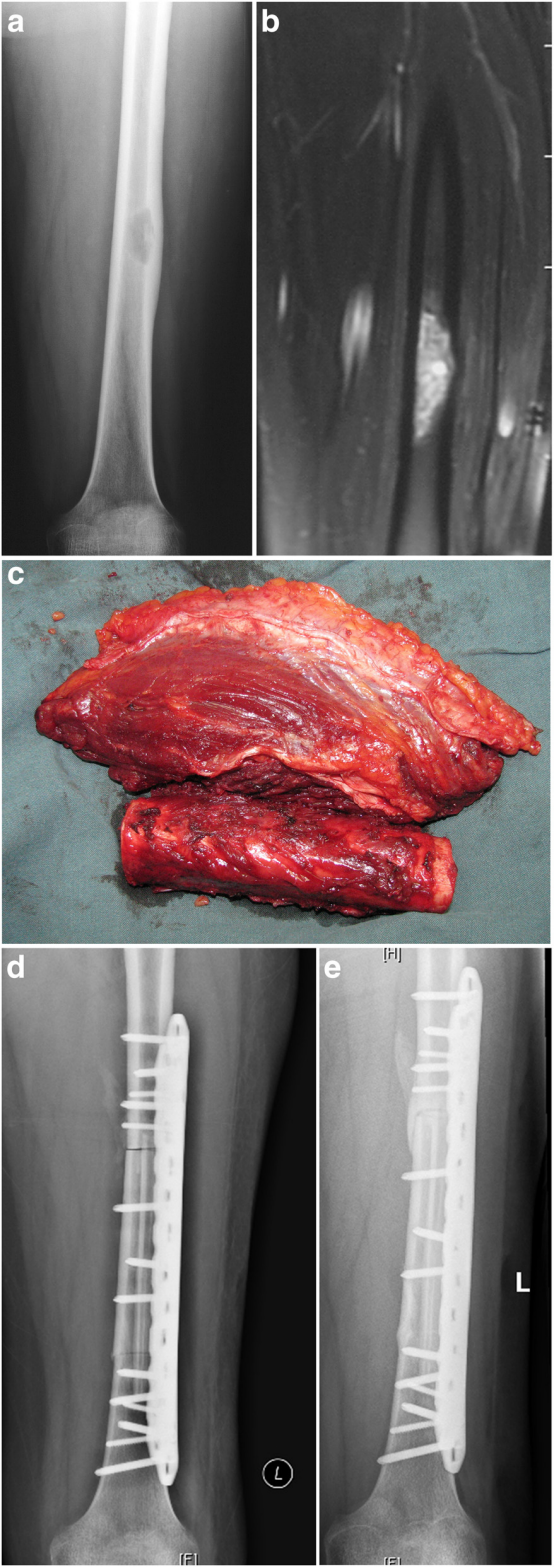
A 26-year-old male patient with an Ewing sarcoma in the middle part of the right femur. a Radiograph showed a lytic lesion in the diaphysial region of the right femur. b MRI showed the lesion was about 8 cm. c A 12-cm segmental bone was resected, the soft tissue was removed, and the structural bone and the periosteum were preserved for divitalization. d Radiograph 2 weeks after the reconstruction showed that the devitalized bone fit the defect perfectly. e Continuous callus at the proximal junction and a perfect union at the distal junction were observed 8 months after the reconstruction
